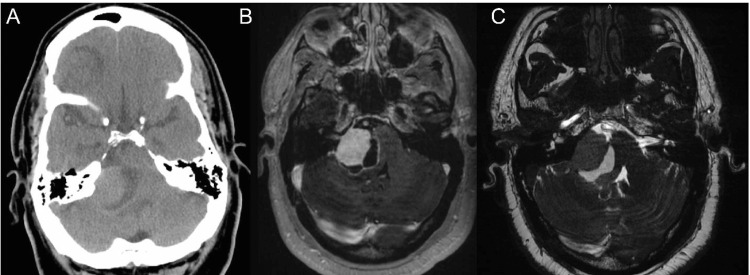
Preoperative images Axial (A) non-contrast CT, (B) contrast-enhanced T1-weighted MRI, and (C) high-resolution 3D FIESTA images Images demonstrate a ~3.5 cm contrast-enhancing mass in the right CPA exerting a mass effect on and displacing the brainstem with effacement of the fourth ventricle. FIESTA: fast imaging employing steady-state acquisition
